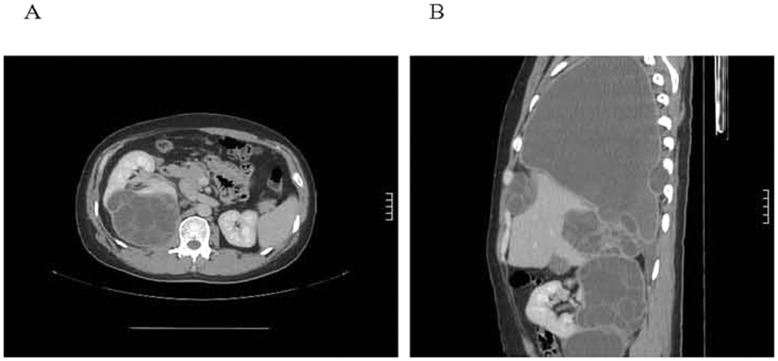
Type CE2 disease in a 35-year-old Han male patient. The patient experienced intermittent fever, shortness of breath, chest tightness, cough, and expectoration of a white-capsule-like substance for one month and aggravation of symptoms for 3 days. He did not experience any itching or palpitation. Physical examination showed dullness on percussion in the right lung and decreased respiratory movement. He underwent two surgeries for hepatic hydatid disease in 1995 and 2003. A CT image showed multiple hydatid cysts in the right lung (A), chest cavity (B), and diaphragm apex. Compression atelectasis of the right lung and a large amount of pleural effusion on the right side was observed. Multiple hepatic hydatid cysts and a renal hydatid cyst with multiple daughter vesicles were also observed.
